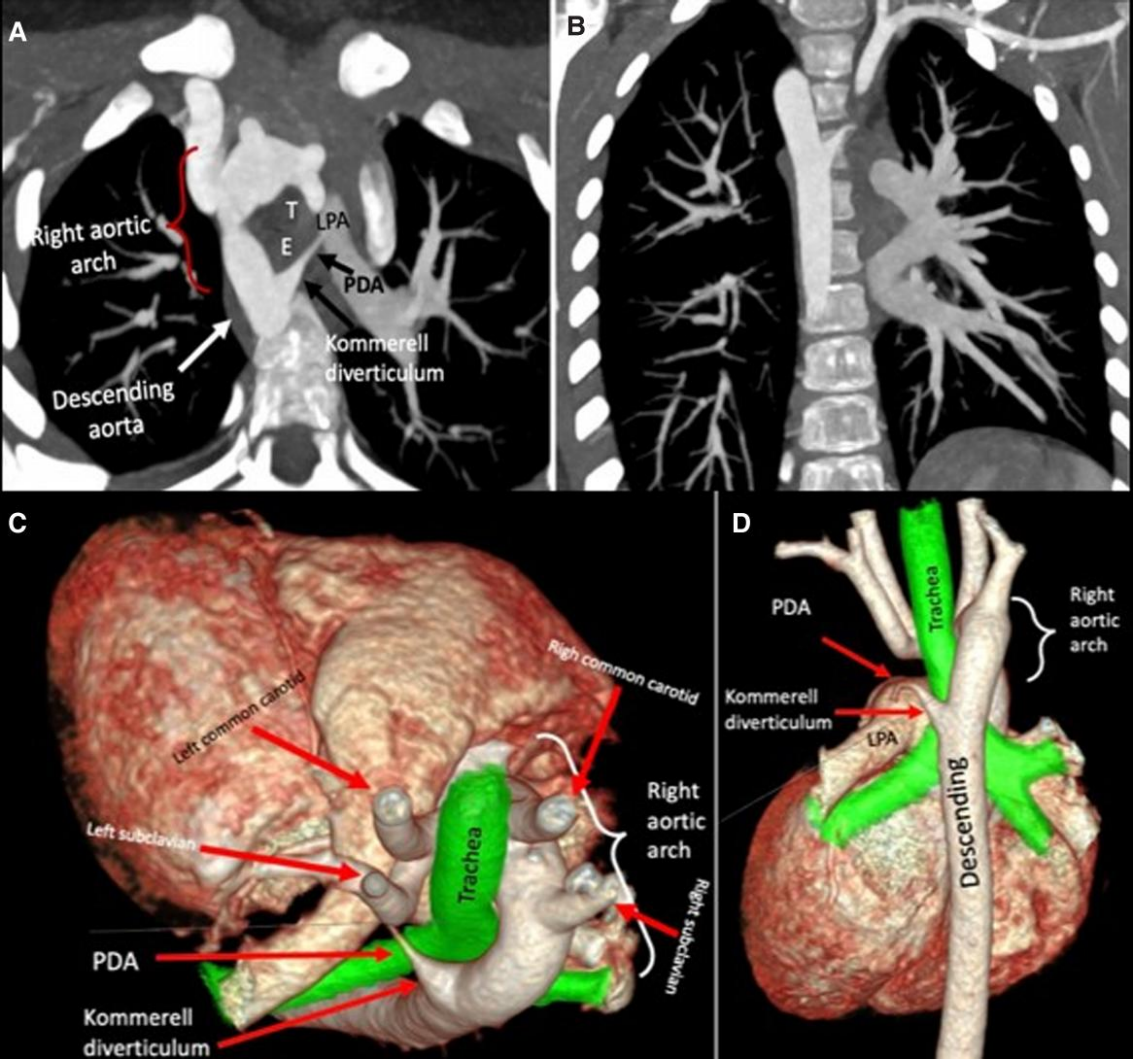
(*A*) The axial oblique reformatted maximum intensity projection image from cardiac computed tomography angiography shows a complete vascular ring made up of a right aortic arch, a left Kommerell diverticulum, and a retroesophageal persistent ductus arteriosus. This anomaly is the result of incomplete regression of the 4th left aortic arch distal to the origin of the left subclavian artery. Abbreviations: LPA, left pulmonary artery; T, trachea; E, oesophagus; PDA, persistent ductus arteriosus. (*B*) Coronal oblique reformatted maximum intensity projection image demonstrates a right ascending aorta with left Kommerell diverticulum. (*C* and *D*) Three-dimensional volume-rendered image in superior and posterior views shows trachea and oesophagus fully surrounded by vascular structures: ascending aorta, right aortic arch, left Kommerell diverticulum, left patent ductus arteriosus, and left pulmonary artery.
